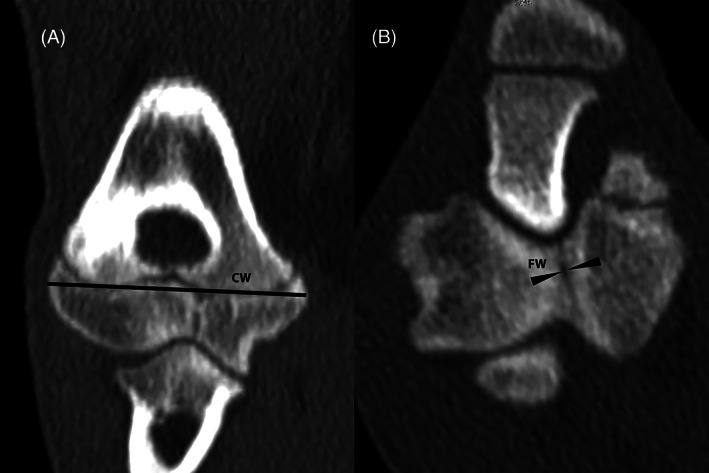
The measurements taken from the contralateral limb CT scan. This includes the condylar width (CW) in the frontal plane (A) and the fissure width (FW) in the transverse plane (B). The CW:FW is then calculated.
